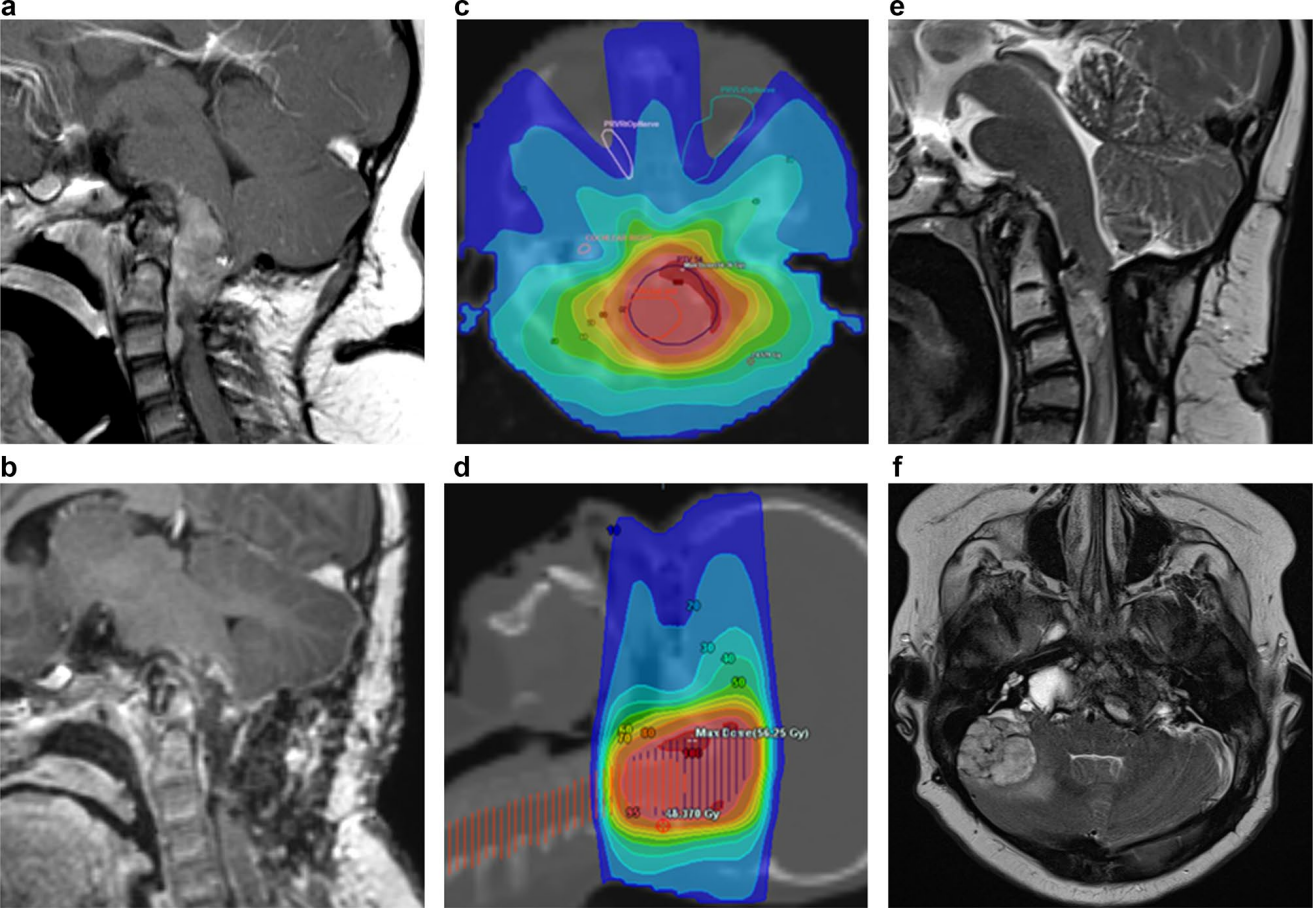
a Post-contrast sagittal T1 image showing enhancing recurrent disease at 12 years of age. b Post contrast sagittal T1 image showing the results of tumour debulking. b–c Fused CT/radiotherapy planning maps. b axial and c sagittal images. d–e Recurrent disease at 13 years of age: d mid-sagittal T2 weighted image demonstrating new cervical disease at C2/3. e Axial T2 weighted image showing extra-axial disease overlying the right cerebellar
